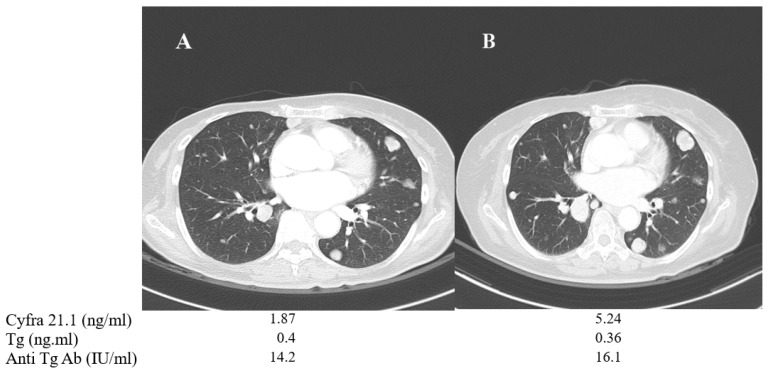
Changes in chest CT images and serum biomarkers of a 57-year-old female with poorly differentiated thyroid cancer (patient No. 1) before (A) and after one year of TKI treatment (B).
